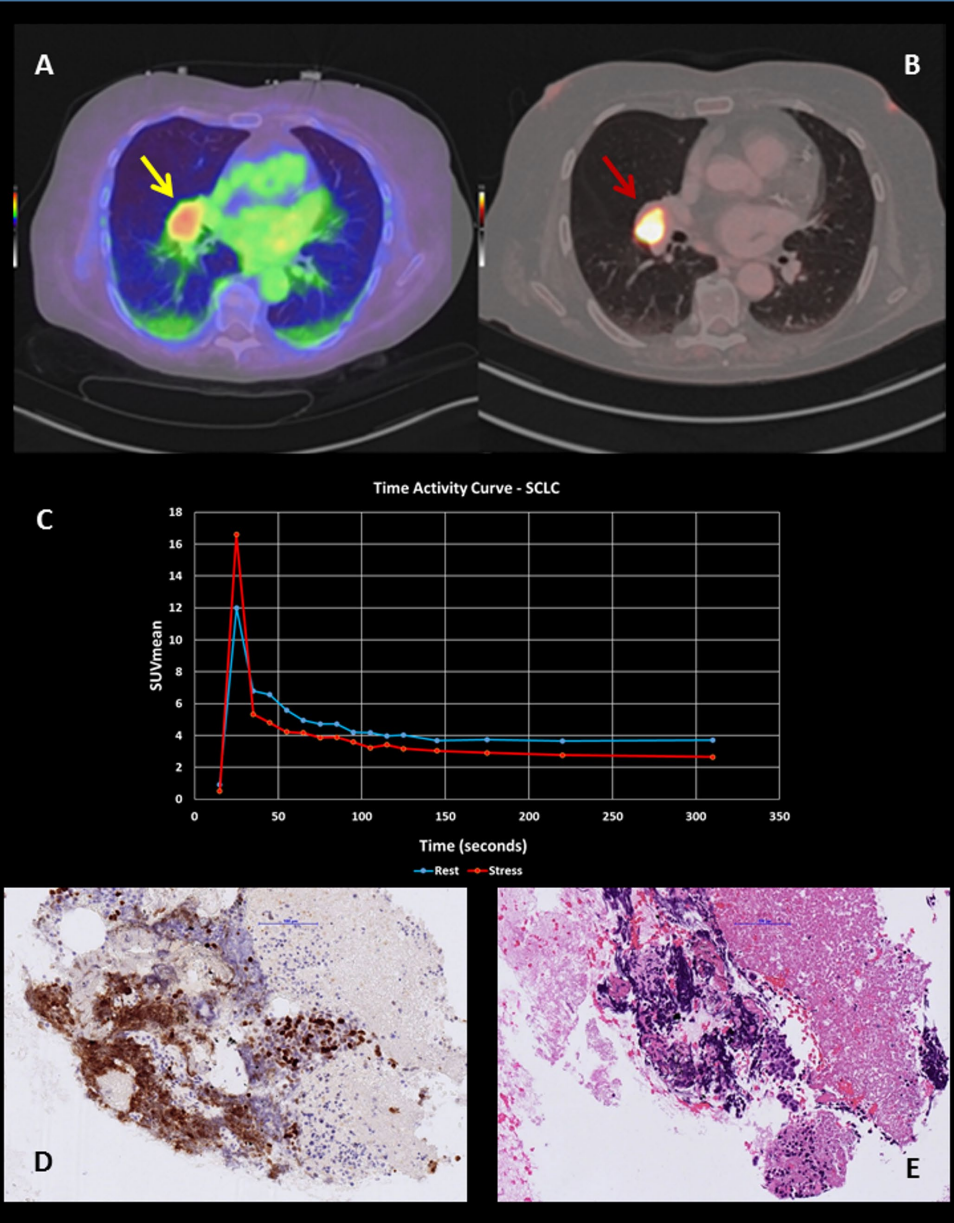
Incidental finding of a hyperperfused right perihilar lung lesion on [^82^Rb]Rb PET/CT performed for myocardial perfusion assessment (A). The lesion demonstrated intense FDG uptake on follow-up [^18^F]FDG PET/CT (B). Dynamic [^82^Rb]Rb PET-derived time-activity curves (C) revealed elevated perfusion under Regadenoson-induced stress. Cytology confirmed small cell lung carcinoma (SCLC) with high proliferative index (Ki-67 >80%) and typical morphology (D, E)
